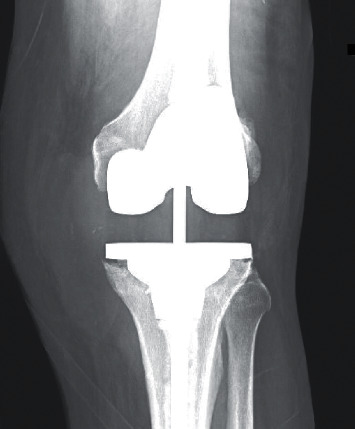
X-ray of the left knee demonstrating perihardware lucencies at the level of the tibial trays.
